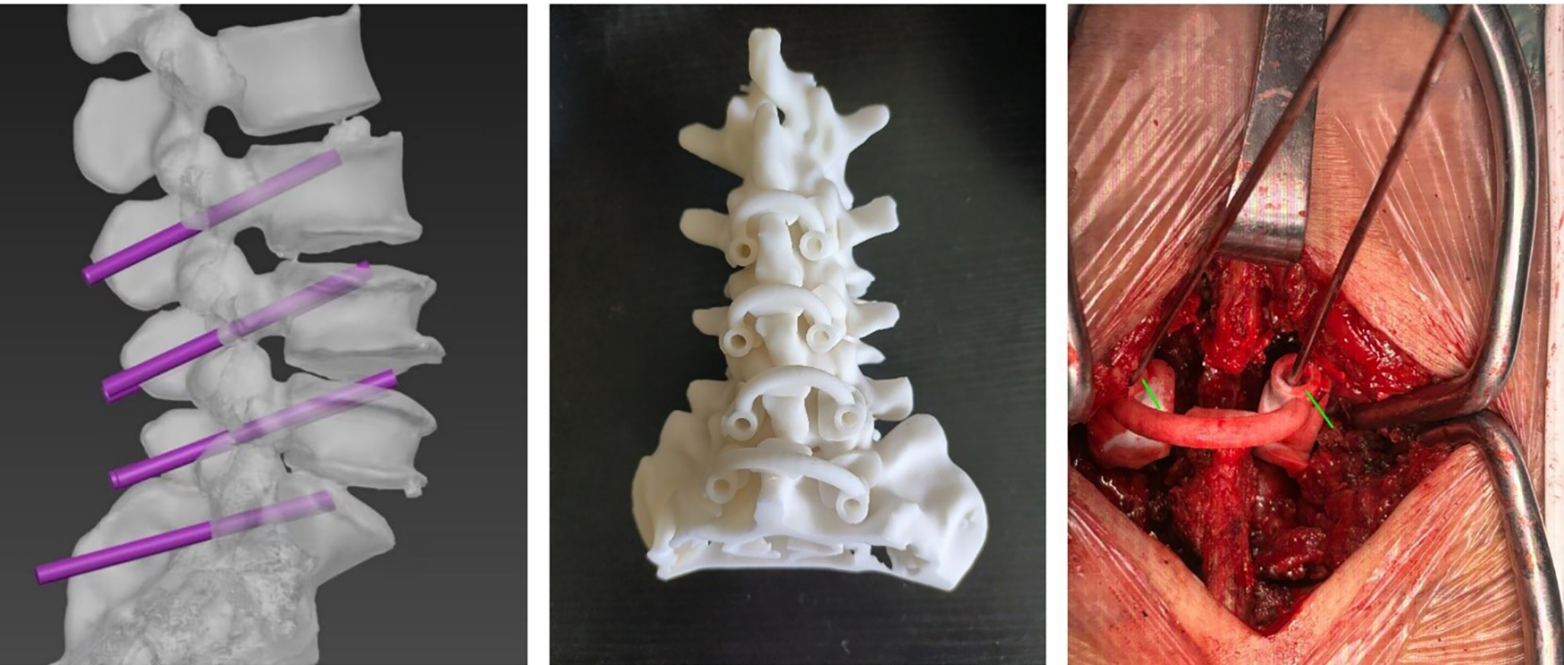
Preoperative and intraoperative steps in lumbar CBT screw implantation using individualized 3D-printing navigation templates. (A) 3D-printing navigation templates created using preoperative lumbar CT data; (B) CBT screw trajectories planned based on CT data; and (C) Intraoperative guidance for lumbar CBT screw implantation using individualized 3D-printing navigation technology.
